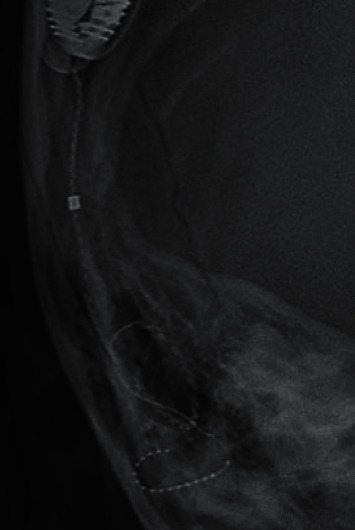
X-ray of the right mastoid (frontal view) showing the implant electrode array possibly coiled up in the region of the external auditory canal.
